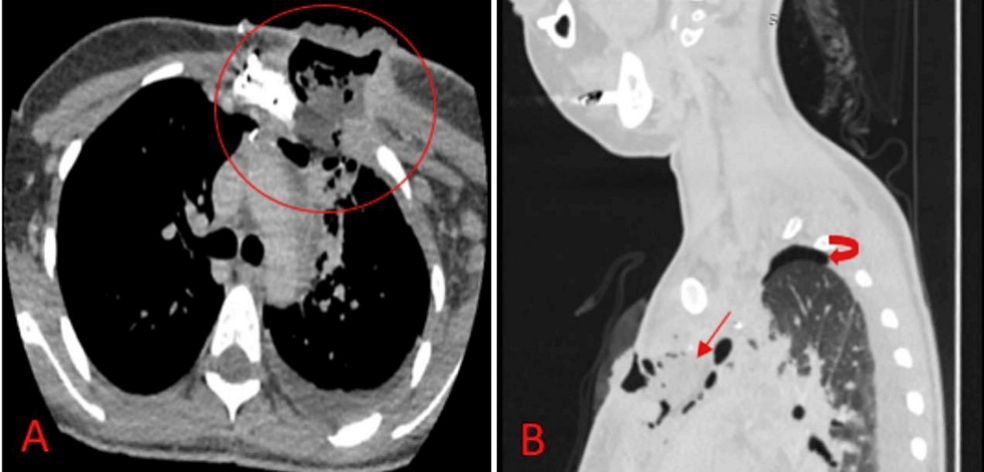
Computed tomography scans of the chest A: the axial mediastinal view showed an infected area around the wound extending deeply into the mediastinum (red circle); B: the sagittal lung view showed the area of suspected infected mass (red arrow), with a pneumomediastinum and a rim of pneumothorax (curved arrow) related to previous surgery.
